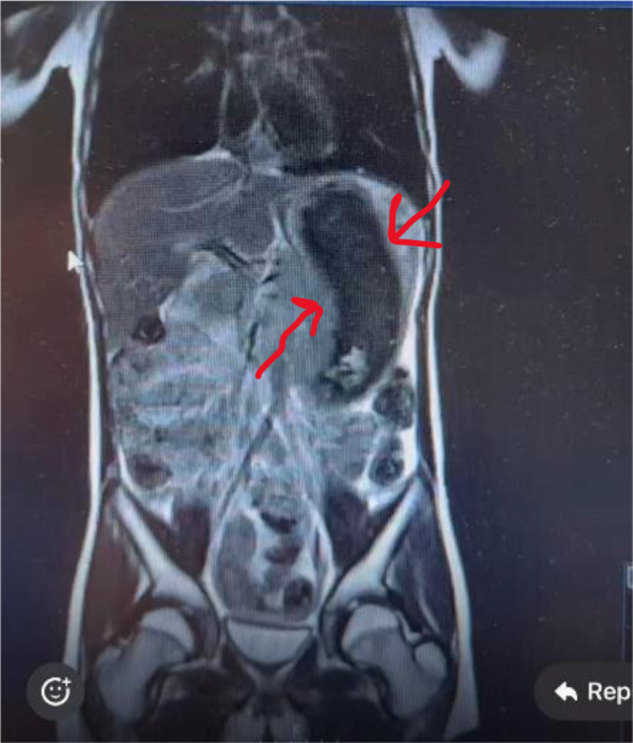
Coronal MRI view shows large TBZ as a well-defined, intragastric mass extending vertically, occupying a significant portion of the gastric lumen. The mass has a heterogeneous signal, suggestive of hair content.
